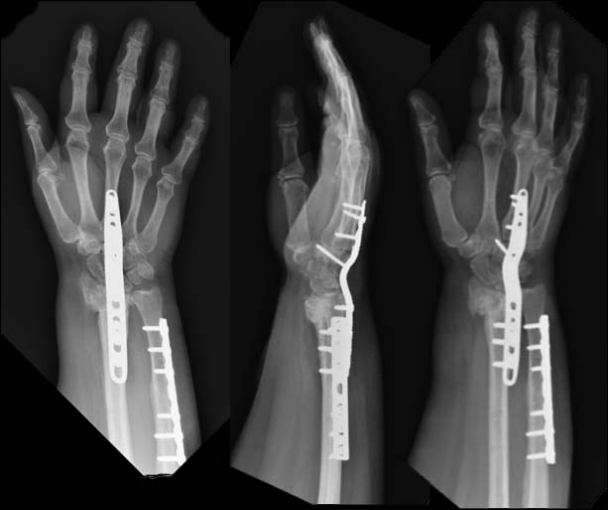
Twelve months after the last surgery, radiographs show consolidation at the nonunion site. Patient at this point is pain-free and has full forearm rotation.
